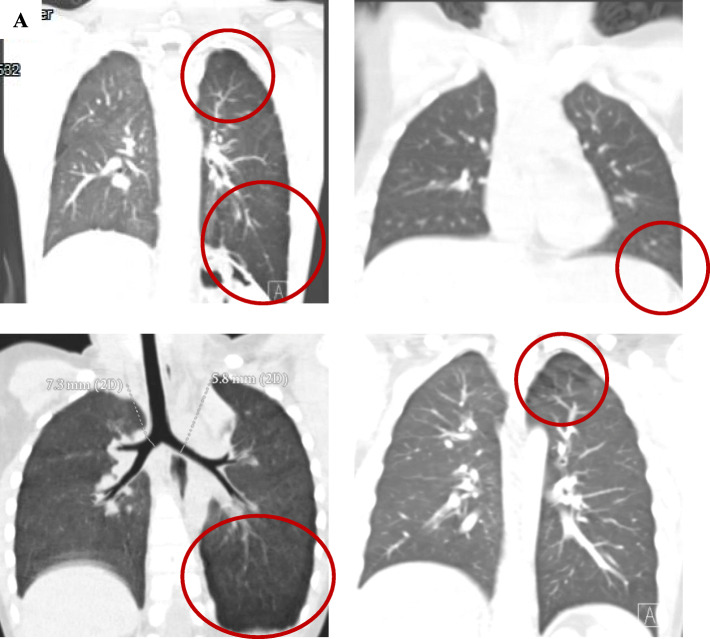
Chest CT scans taken in children with repaired congenital diaphragmatic hernia. Note the multiple emphysematous lesions of the affected lungs in the apical and basal segments (red circles)
